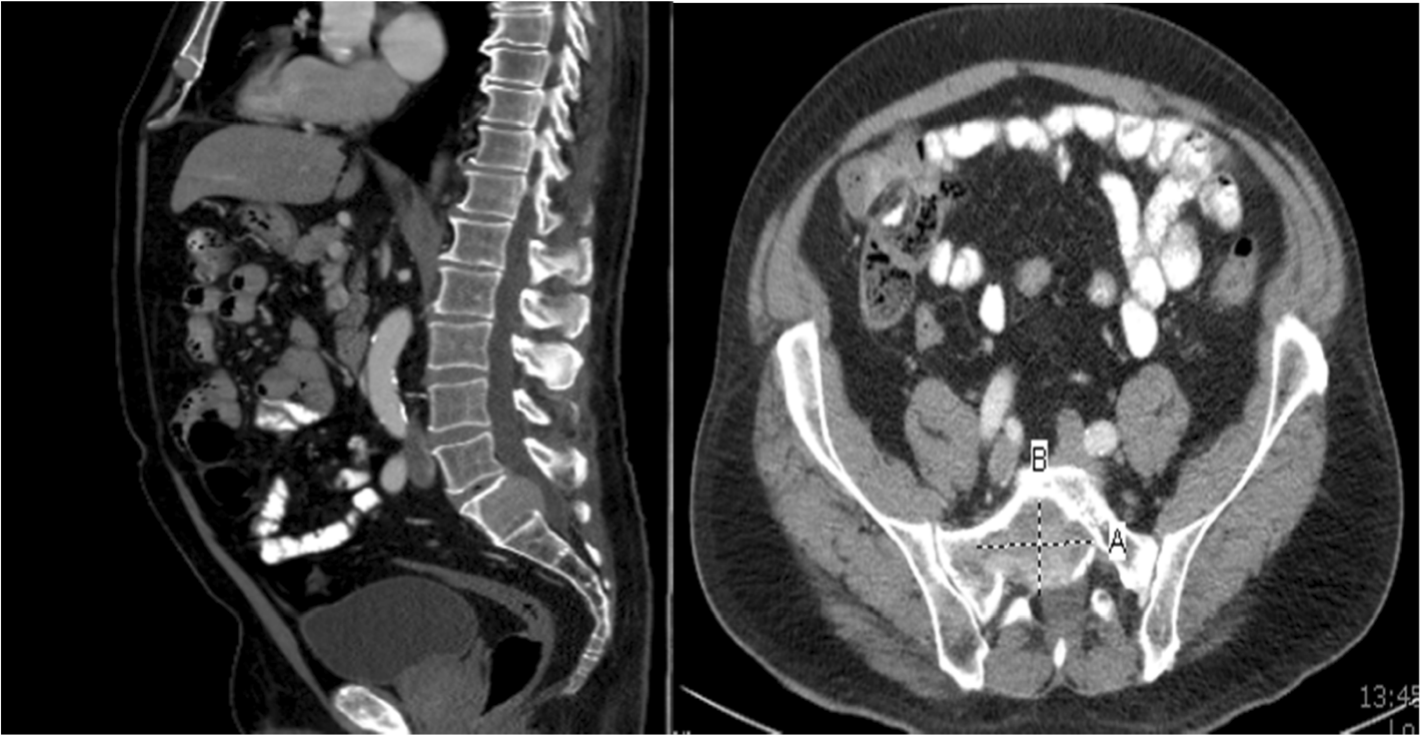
Comparison of Sacral Metastasis Showing No Progression after Treatment with Pembrolizumab and SBRT, (Left) CT abdomen/pelvis sagittal view from 9/22/2017 revealing metastasis in the S1 vertebral body extending posteriorly into the spinal canal. (Right) CT abdomen/pelvis axial view from 12/14/2017 showing unchanged size of sacral metastasis
